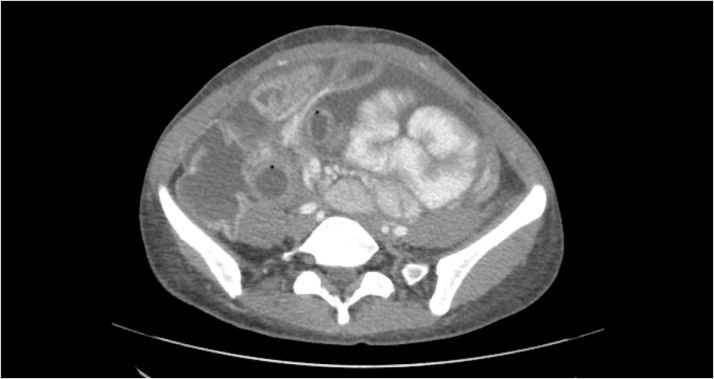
CT from March 1, 2018 shows extensive ascites with small bowel clumped from mesenteric retraction by cancer.
